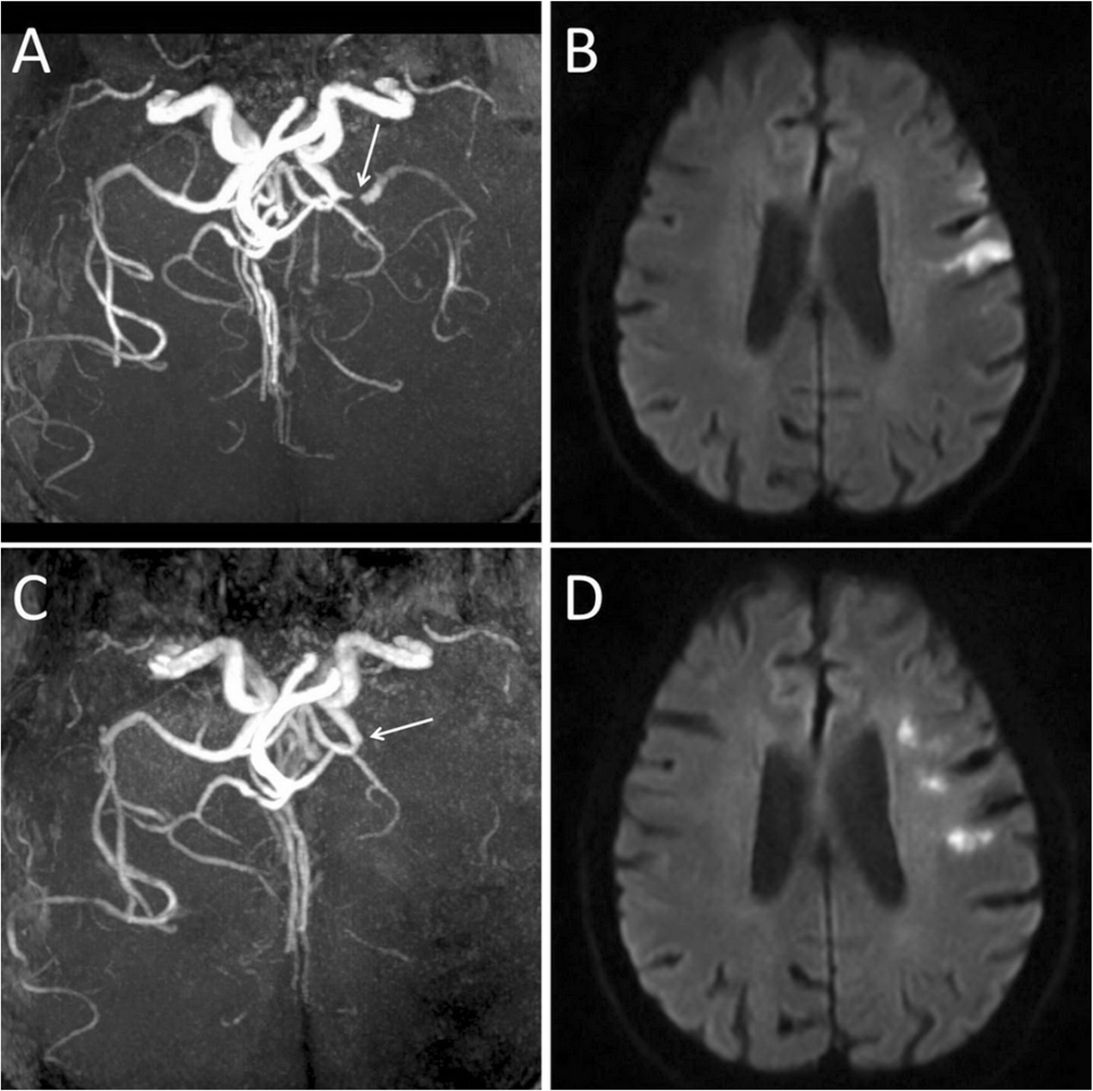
A case of stroke recurrence. On the third day after stroke onset, MRA (a) shows a severe stenosis of the left MCA trunk (white arrow) and a severely reduced signal intensity of the left MCA branches distal to the stenosis (grade C); DWI (b) shows acute cortical infarctions of the left frontal lobe. This patient experienced a recurrent stroke on the 71st day after the initial onset. On the 73rd day after the initial onset, MRA (c) shows a proximal occlusion of the left MCA trunk (white arrow); DWI (d) shows acute cortical and subcortical infarctions of the left frontal lobe
